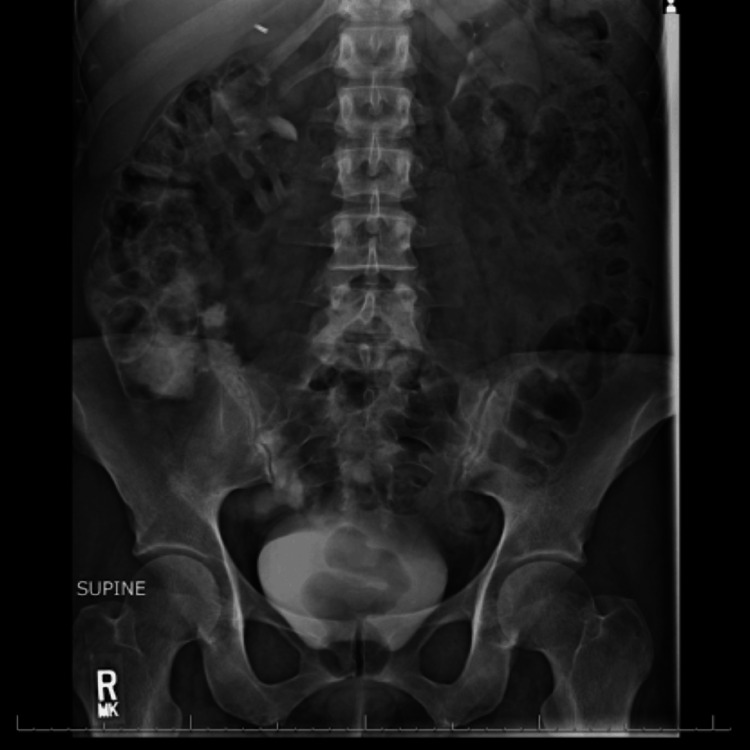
Supine abdominal radiograph showing moderate colonic stool burden
